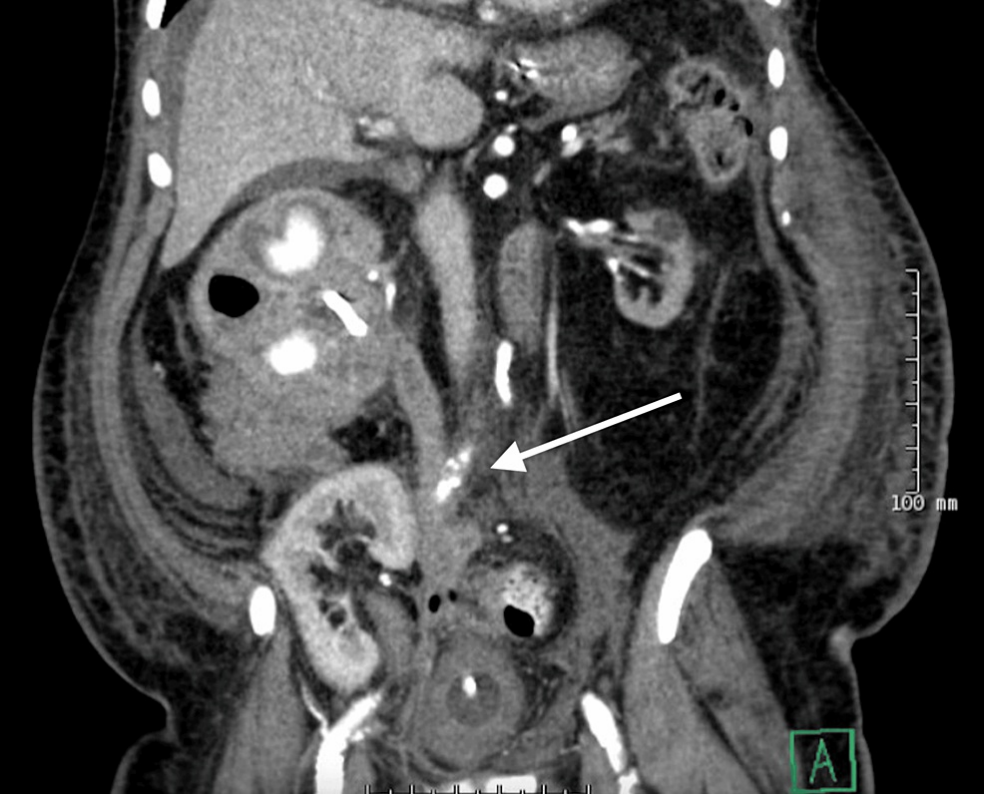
CT of the abdomen and pelvis with findings concerning for a focal sigmoid colon perforation with a right ureterocolic fistula involving the right native ureter
